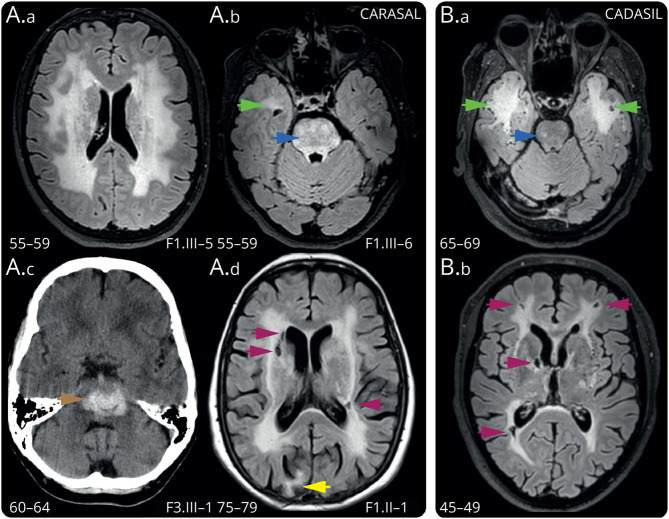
Neuroimaging Abnormalities in Patients With CARASAL To ensure patient anonymity, only age ranges are given. (A.a.) T2-FLAIR: a 55–59-year-old female patient with CARASAL with confluent WMHs. This patient had no lacunes, and only 5 CMBs were present in the pons (not shown). (A.b.) T2-FLAIR: a 55–59-year-old female patient with CARASAL with striking WMHs in the pons (blue arrowhead) and unilateral WMHs in the right ATL (green arrowhead). (A.c.) CT-scan: a 60–64-year-old male patient with CARASAL with a fatal ICH located in the pons (brown arrowhead). (A.d.) T2-FLAIR: a 75–79-year-old female patient with CARASAL with 3 lacunes (purple arrowheads) and a cortical infarct in the occipital lobe (yellow arrowhead). (B.a.) T2-FLAIR: a 65–69-year-old male patient with CADASIL with confluent WMHs in the ATL, with associated juxtacortical perivascular spaces (green arrowheads). This patient had the highest nWMHv in the DiViNAS cohort. Note that there are only a few punctate foci of WMHs present in the pons (blue arrowhead). (B.b.) T2-FLAIR: a 45–49-year-old male patient with CADASIL with extensive WMHs and multiple lacunes (purple arrowheads). ATL = anterior temporal lobe; CADASIL = cerebral autosomal dominant arteriopathy with subcortical infarcts and leukoencephalopathy; CARASAL = cathepsin A–related arteriopathy with strokes and leukoencephalopathy; DiViNAS = Disease Variability in NOTCH3-associated Small Vessel Disease; FLAIR = fluid-attenuated inverse recovery; nWMHv = normalized white matter hyperintensity volume; WMHs = white matter hyperintensities.
